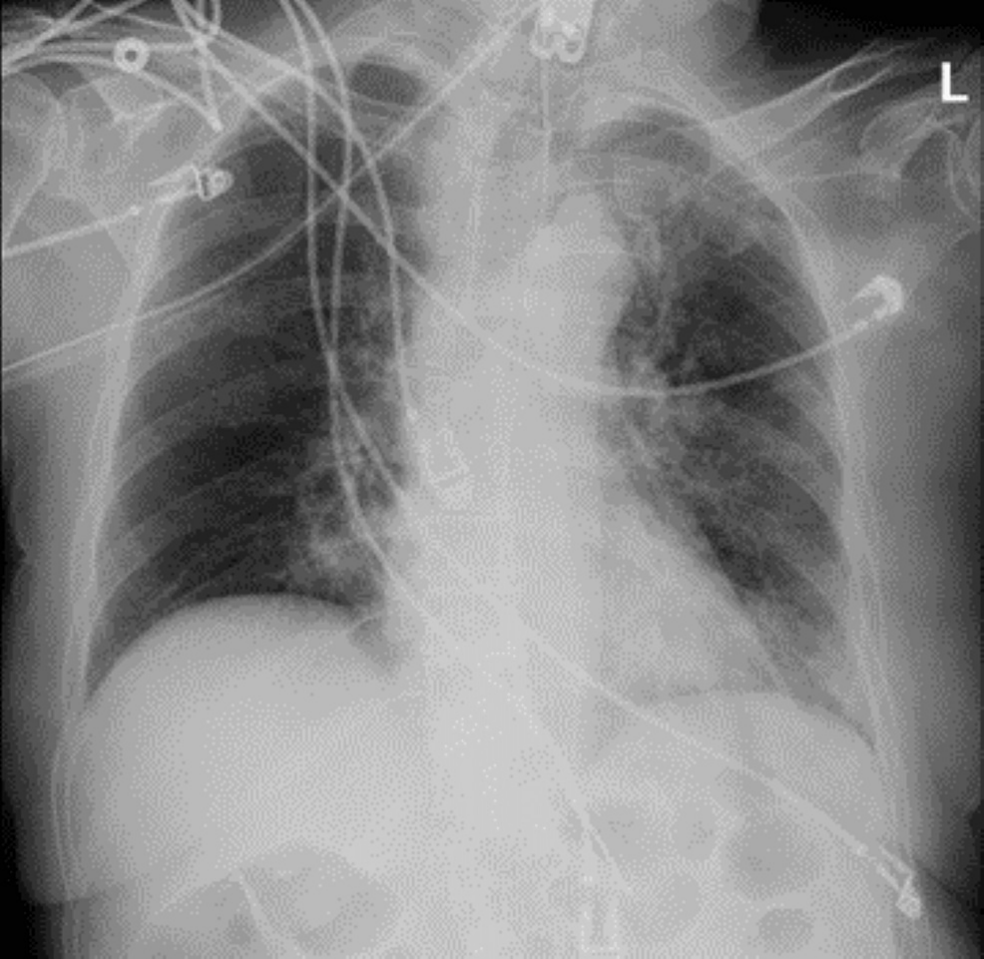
CXR showing improvement of left lower lobe pneumonia. CXR: Chest X-ray
